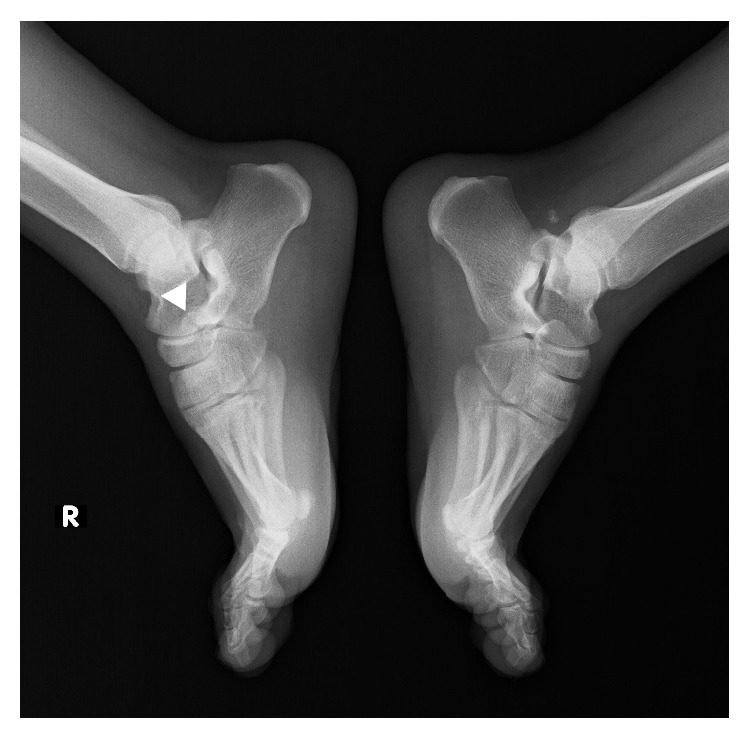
Lateral plain radiograph shows a suspicious fracture line of anterosuperior calcaneal process (white arrowhead).
